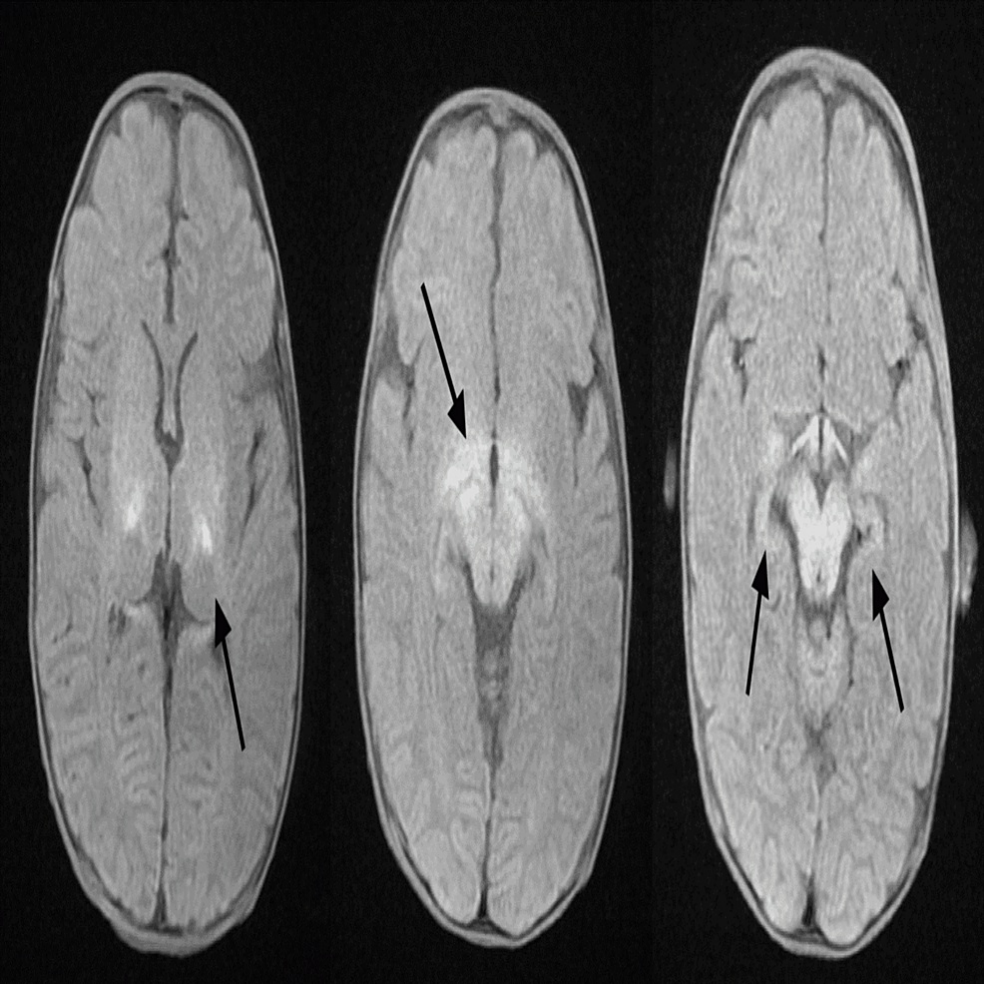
MRI of the brain showing abnormal signal intensity involving the ventral lateral thalami and subthalamic nuclei which was compatible with hyperglycemic encephalopathy (see black arrows).
